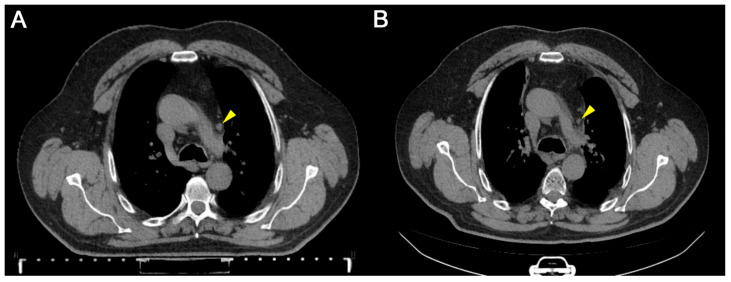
Computed tomography of the chest, axial section, the prevascular lymph node. (A): Before the treatment by immunotherapy with autologous activated lymphocytes in combination with tomotherapy. (B): Three months after the treatment. Lymph node is denoted by a yellow pointer; in dynamics, a substantial decrease in size of the lymph node is noted.
